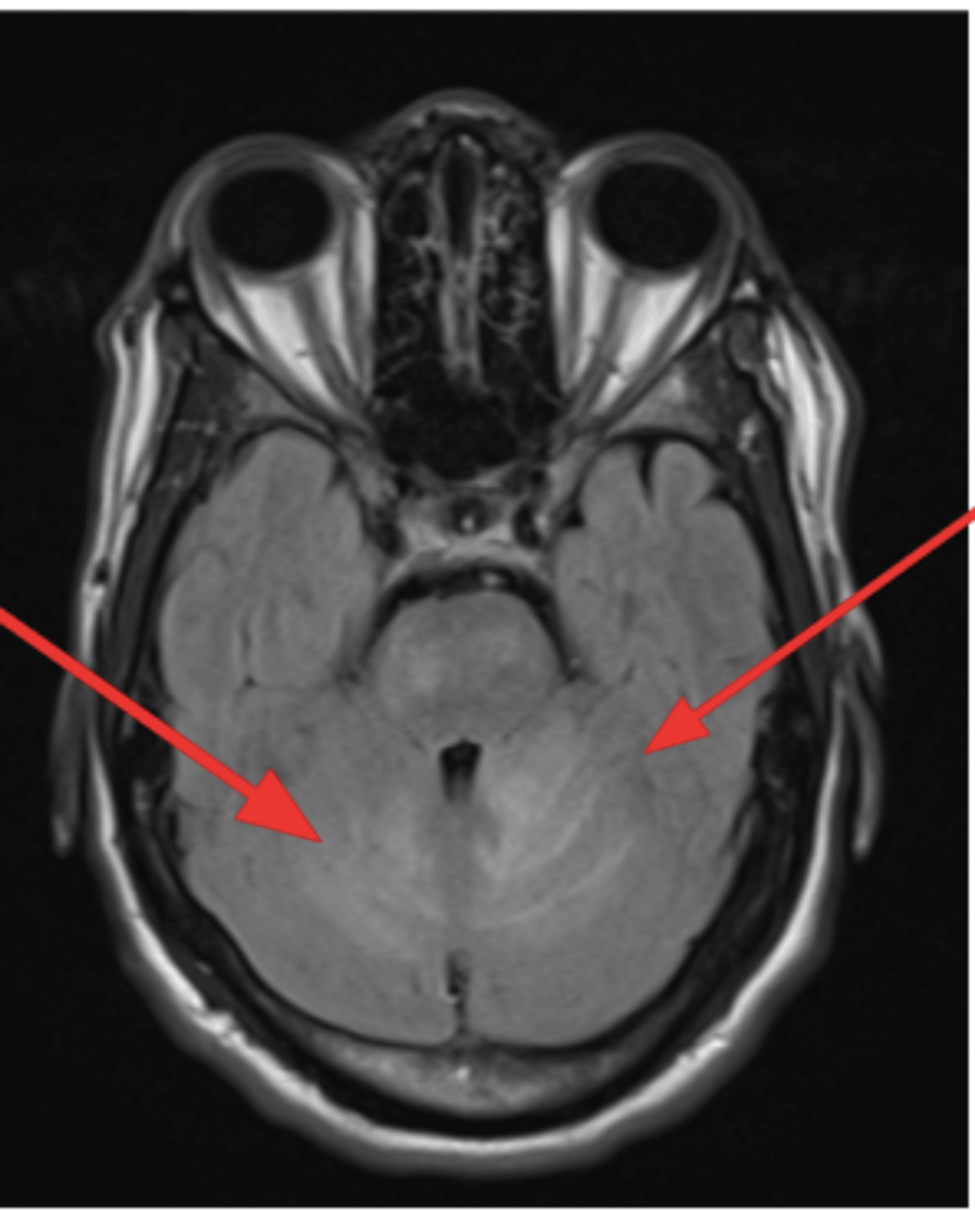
MRI of the brain T2-FLAIR sequence (axial view) MRI of the brain T2-FLAIR sequence showed a decrease in the size of the cerebellar hyperintense area, representing an improvement in cerebellar edema compared with Figure 4 (red arrows). T2-FLAIR: T2-weighted Fluid-Attenuated Inversion Recovery
